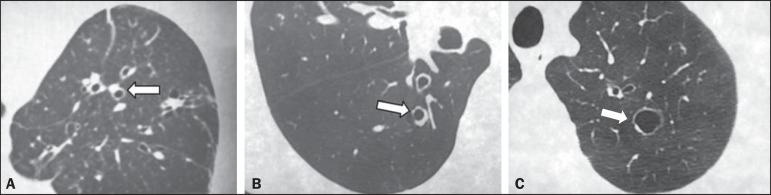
Chest HRCT scans of patients with cylindrical bronchiectasis (arrows).
